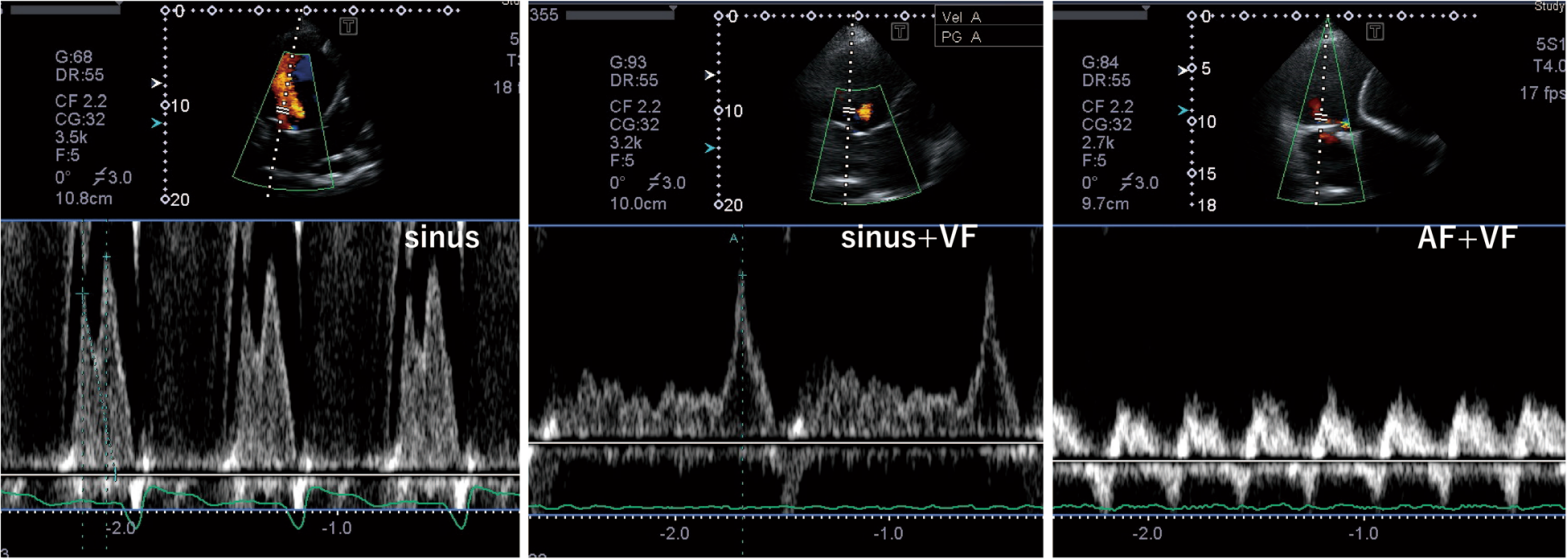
Pulse-wave Doppler showing transmitral flow wave in each rhythm. Normal mitral valve E and A waves were observed in sinus rhythm, whereas only mitral valve A wave could be observed during sinus rhythm and ventricular fibrillation (sinus + VF). During atrial and ventricular fibrillation (AF + VF), no obvious E or A waves were observed; however, small fibrillating waves were detected. Abbreviations: AF: atrial fibrillation, VF: ventricular fibrillation
